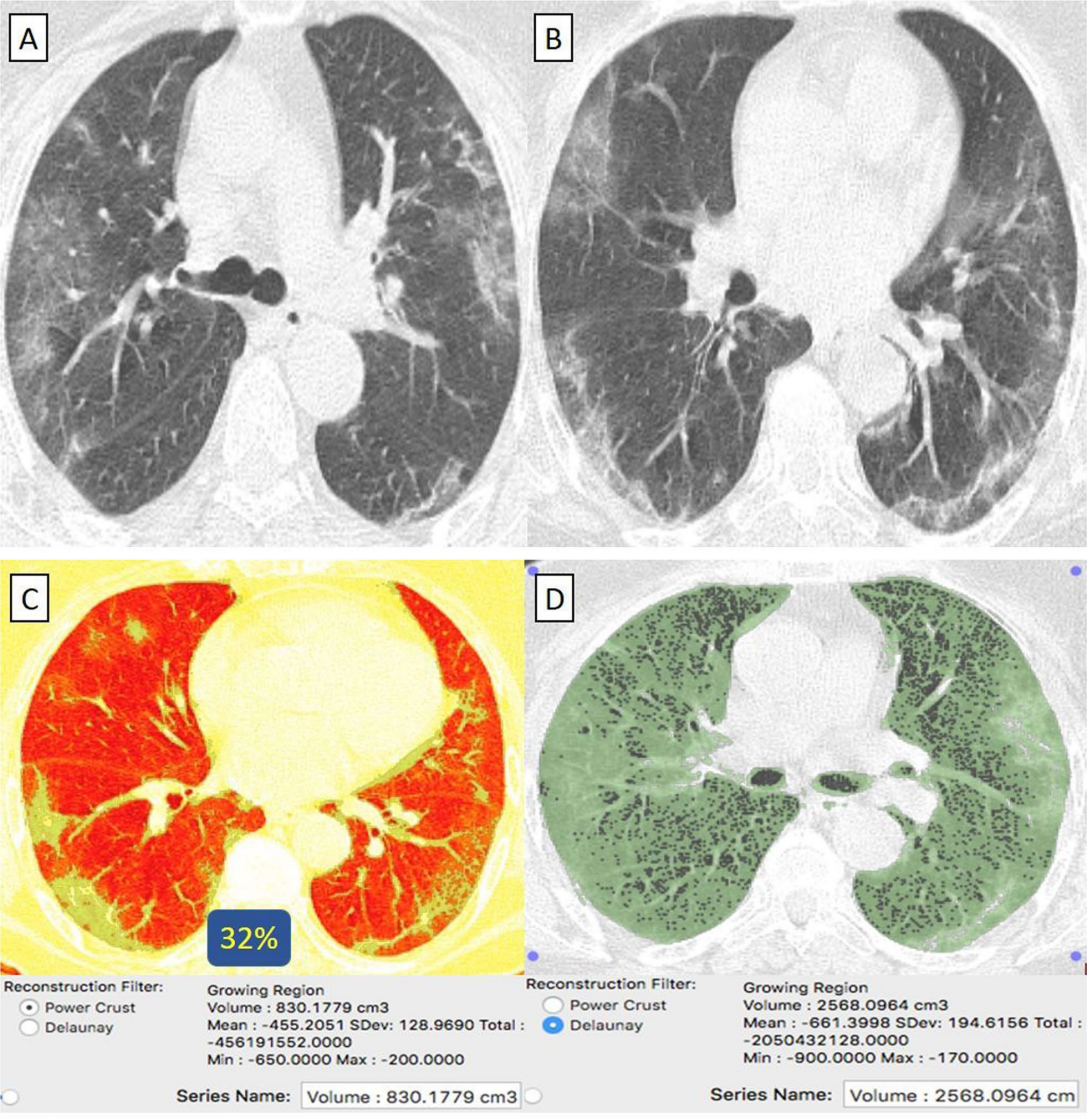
A 55-year-old female patient with negative past medical history and had received “Sinopharm” vaccine then got COVID-19 infection. A, B Axial chest CT cuts (lung window) showed bilateral mainly peripheral located ground-glass pneumonic patches with peripheral sub-pleural sparing (Atol-sign). C CT-Volumetry of the pathological lung parts averages 830 cc. D CT-Volumetry of total lung volume averages 2568 cc. So, 32% of the lung parenchyma was diseased (Score 2)
